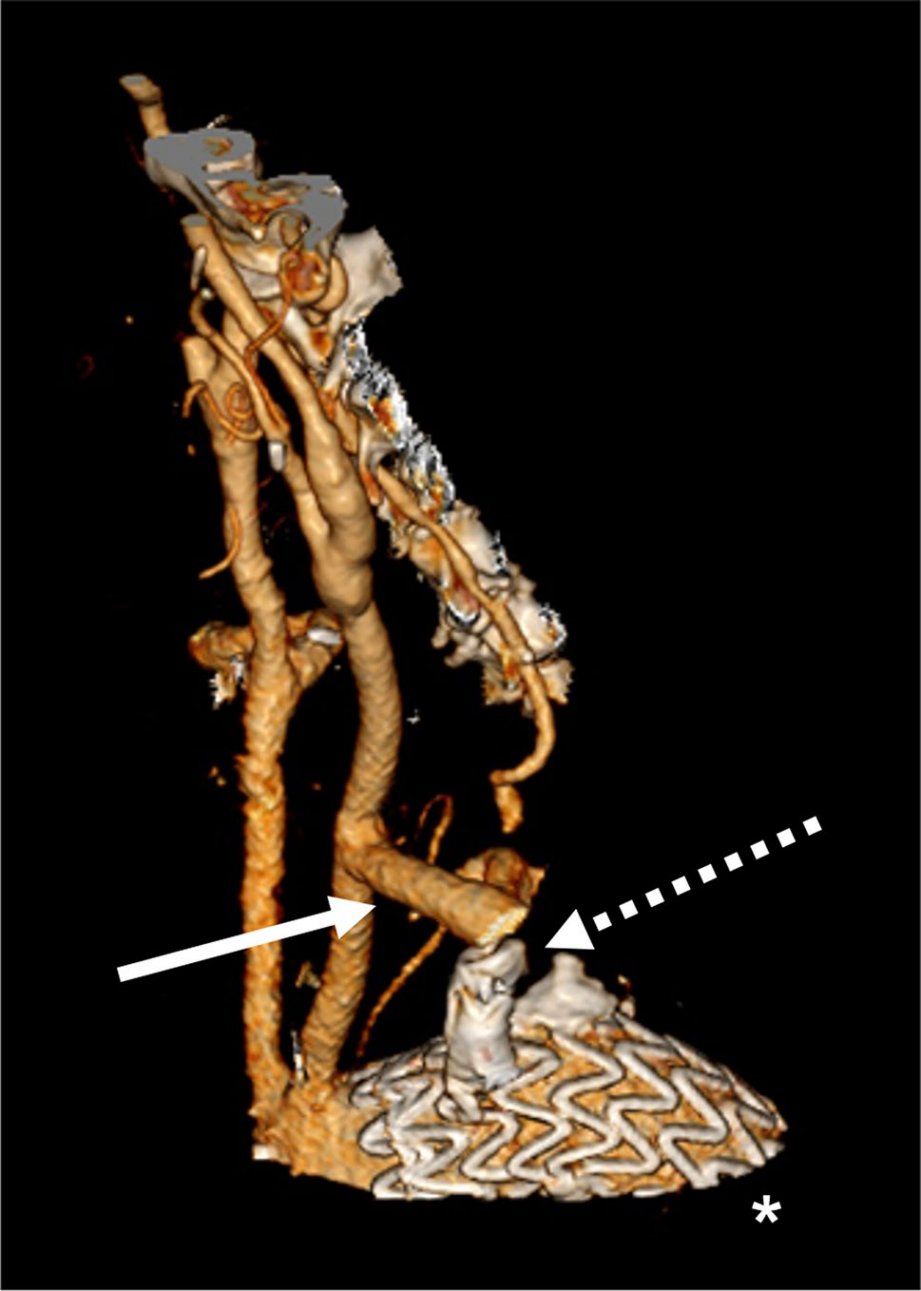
3D reconstruction of the postoperative CT (computed tomography) angiography. The thoracic stent prosthesis (*), the vascular plug implanted in the left proximal subclavian artery (dashed arrow) and the bypass between the common carotid artery and the subclavian artery (arrow) are shown. In addition, the high-grade stenosis of the left vertebral artery can be seen
